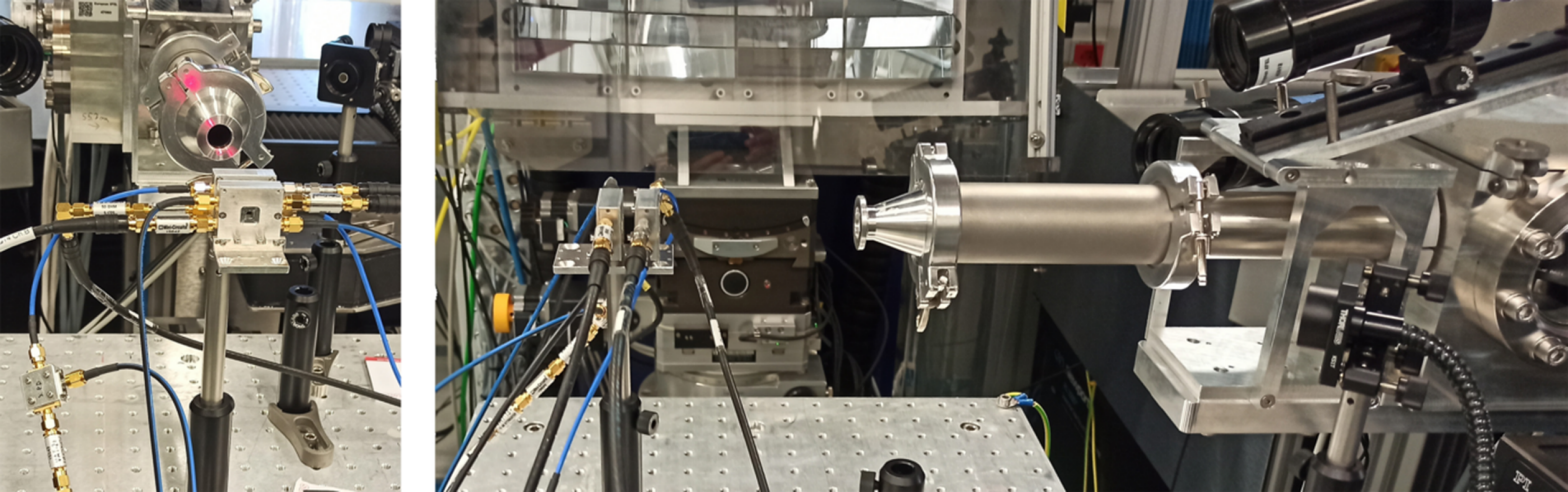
Two different views of the setup at the FXE Hutch to test the new diamond detectors. The X-ray beam propagates to both detectors through the He path exit apperture (round KF flange).
